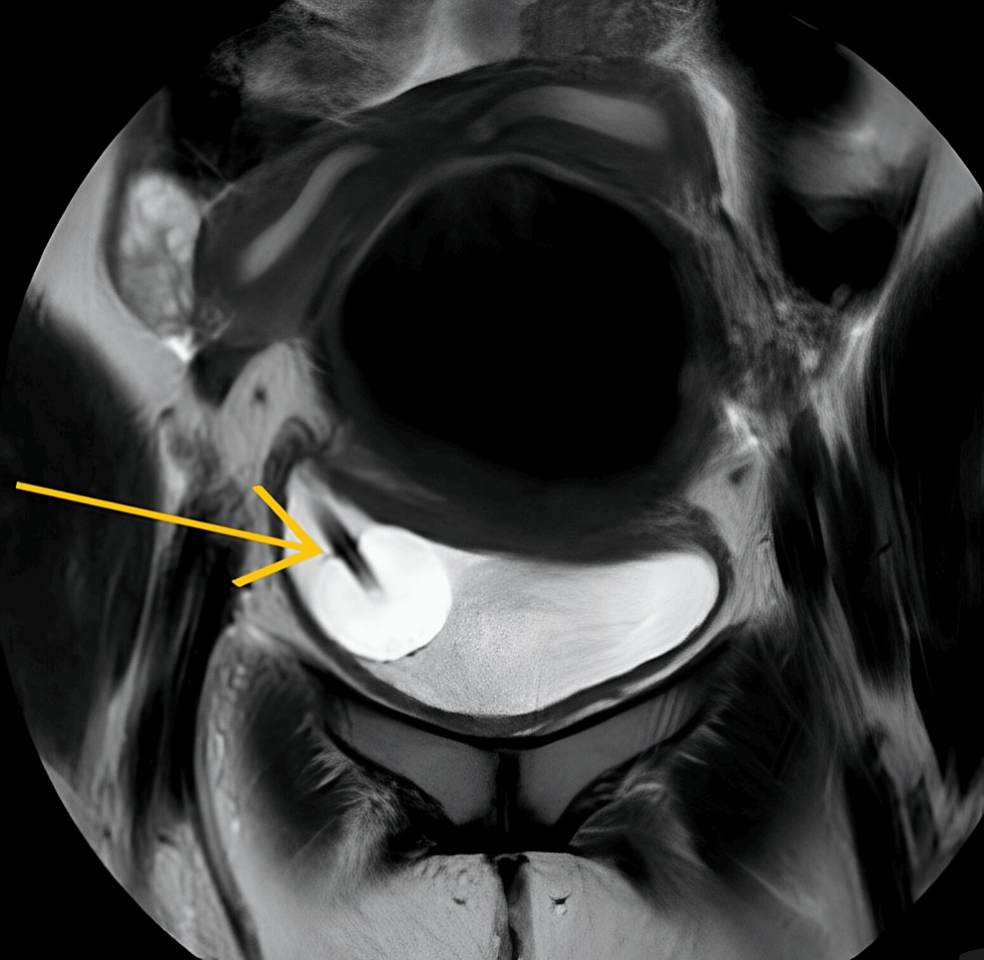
MRI showing uterus with two horns suggestive of bicornuate uterus MRI: magnetic resonance imaging
